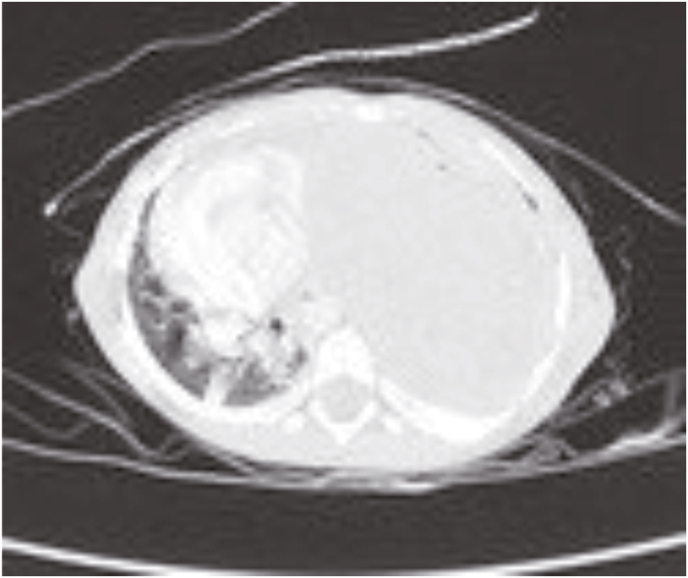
Pleuropulmonary blastoma type 2 (mixed) caused the mediastinum to shift to the right in thorax CT-scan.
